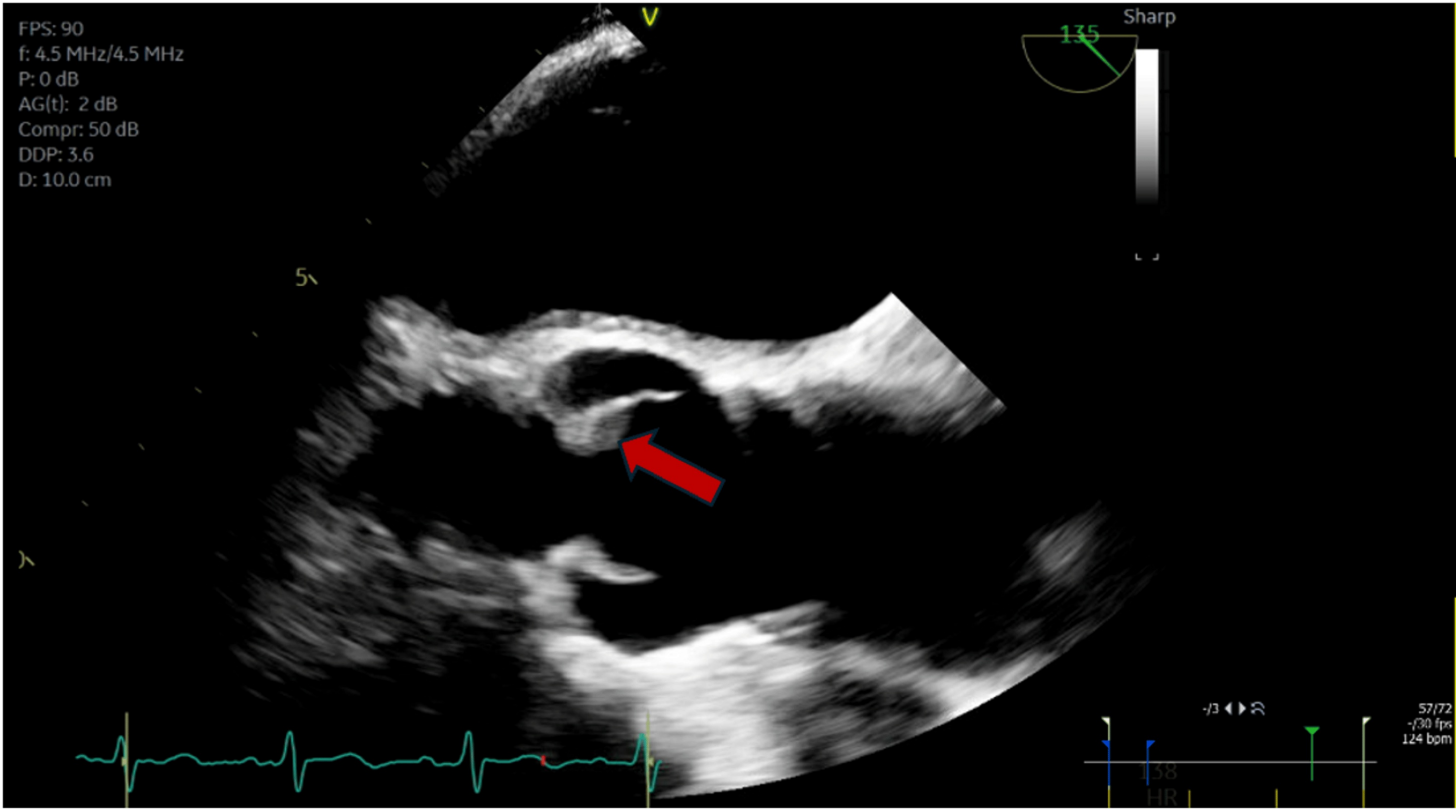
TEE showing a 6-mm fixed lesion (red arrow) on the ventricular aspect of the noncoronary cusp of the aortic valve The presence of this echocardiographic finding in the setting of bacteremia raised the suspicion of infective endocarditis, for which the patient was continued on a prolonged course of ceftriaxone. The echocardiographic finding persisted despite the antibiotic therapy and patient clinical improvement. It was labeled as a valve thickening on a follow-up echocardiography read TEE: transesophageal echocardiography
